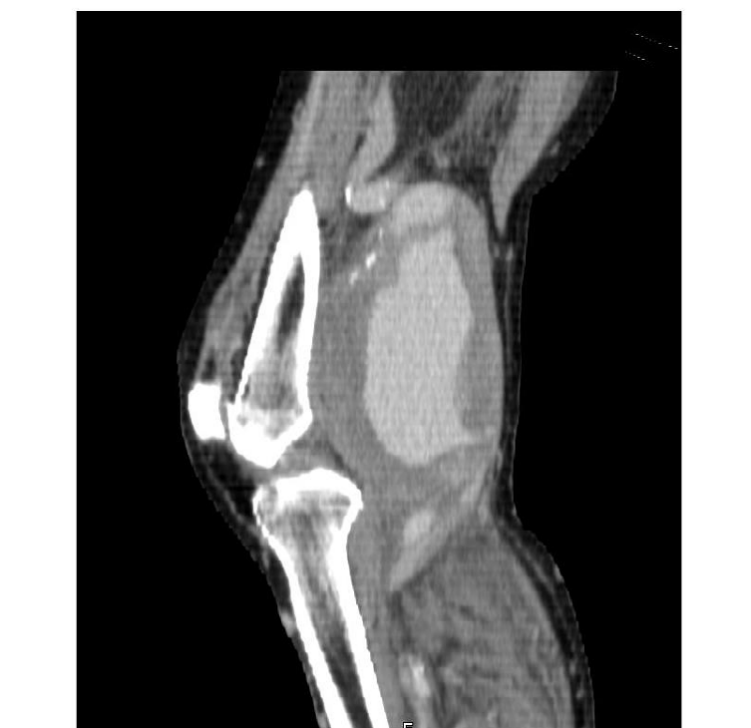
CT lateral projection showing the popliteal artery aneurysm with the pseudoaneurysm.
